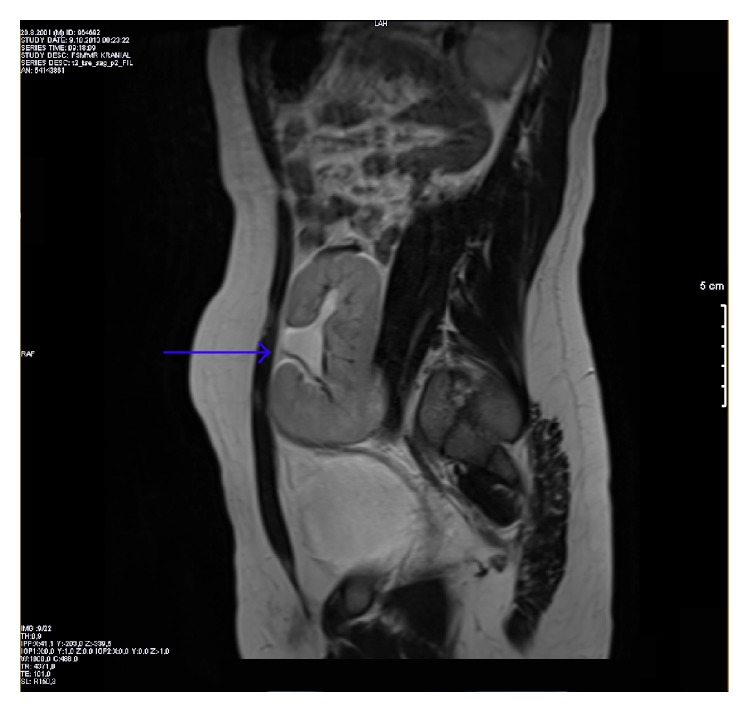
Magnetic resonance imaging of abdomen on the sagittal plane. The left kidney that had one ureter was located over the urinary bladder (blue arrow).
